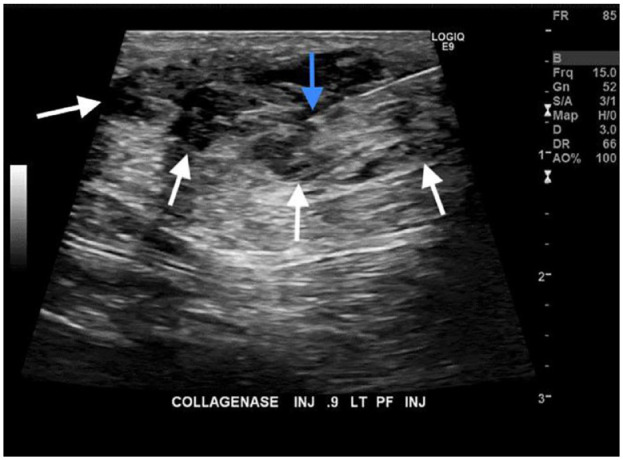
Ultrasonographic image during ultrasonographically-guided fine needle placement (needle tip: blue arrow) into the center of the mass (mass outlined by white arrows) and injection of collagenase (Xiaflex) into the plantar fibroma.
